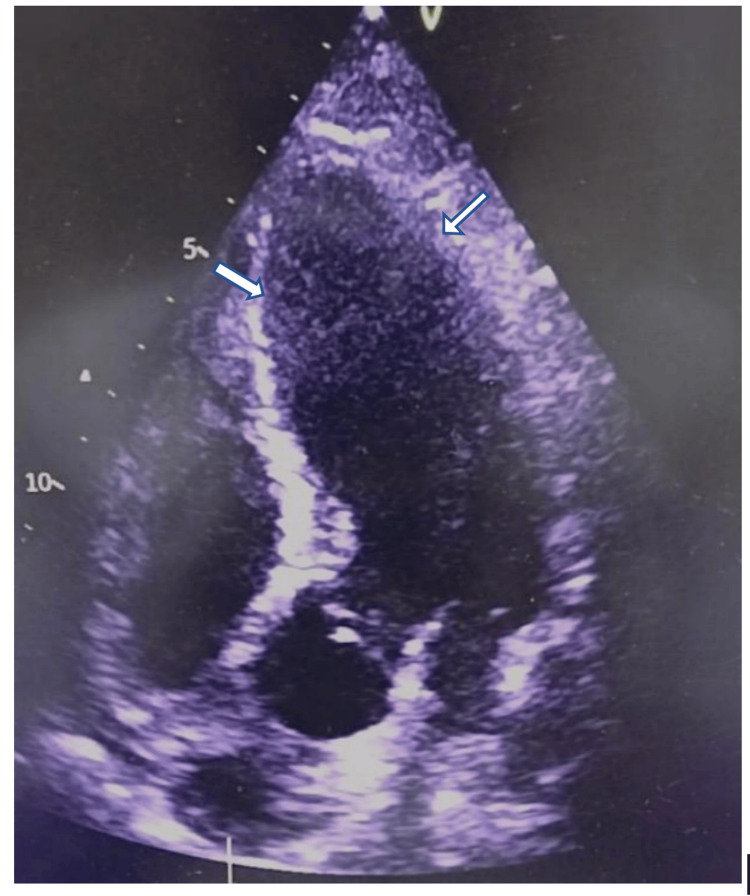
Echocardiography of the heart (apical view) shows a mildly dilated left ventricle with an ejection fraction of 20-25% (white arrow)
